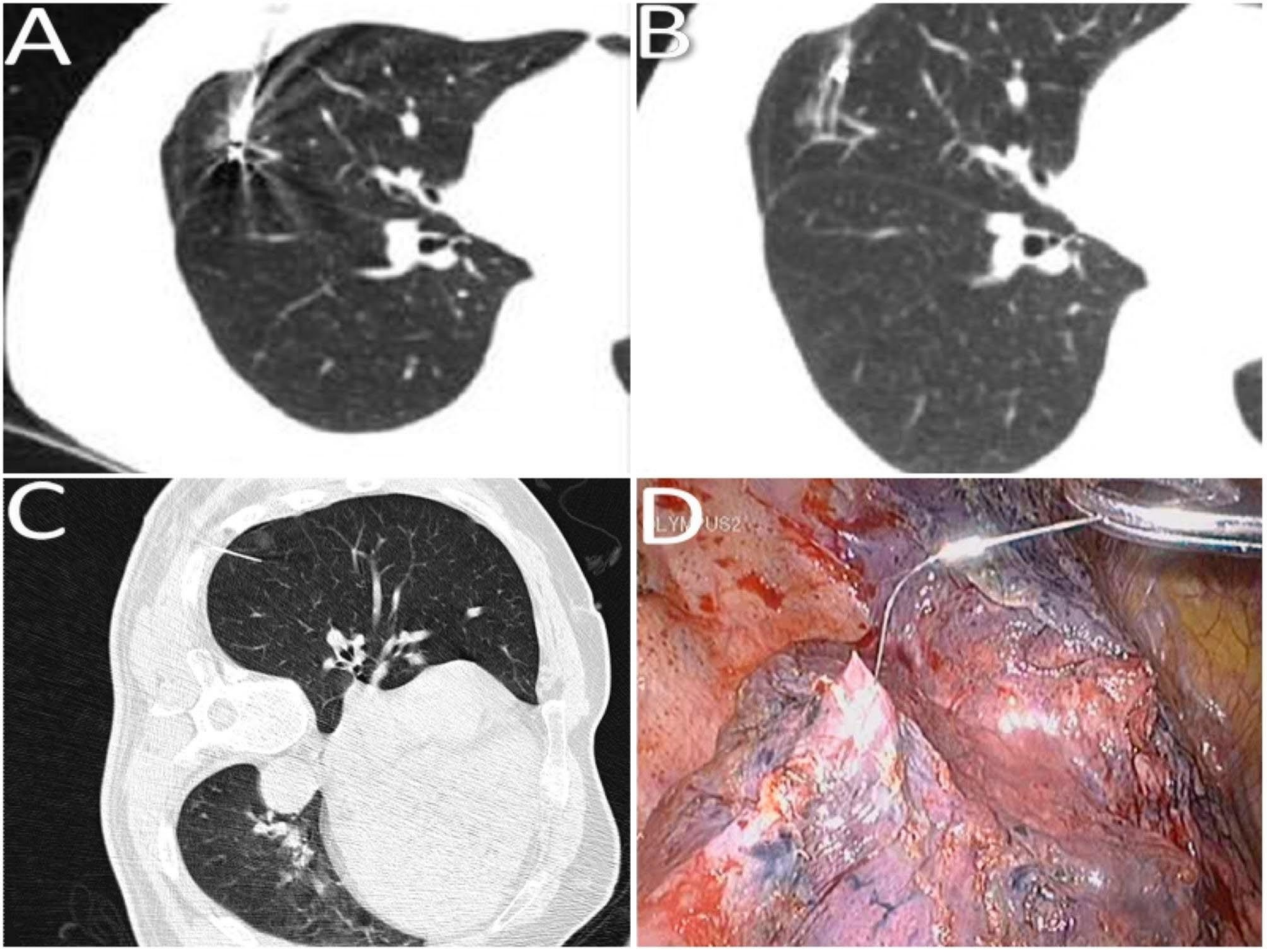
Images of lung computed tomography (CT) scan and surgical field for dislodgement of marking materials. (A) The coil was adjacent to a GGN after it was released. (B) Coil dislodgement occurred through the needle passage. (C) The tip of the hookwire was extended more than 3 cm into the lung tissue before the operation. (D) The tip of the hookwire was extended less than 1 cm into the lung tissue during an operation
